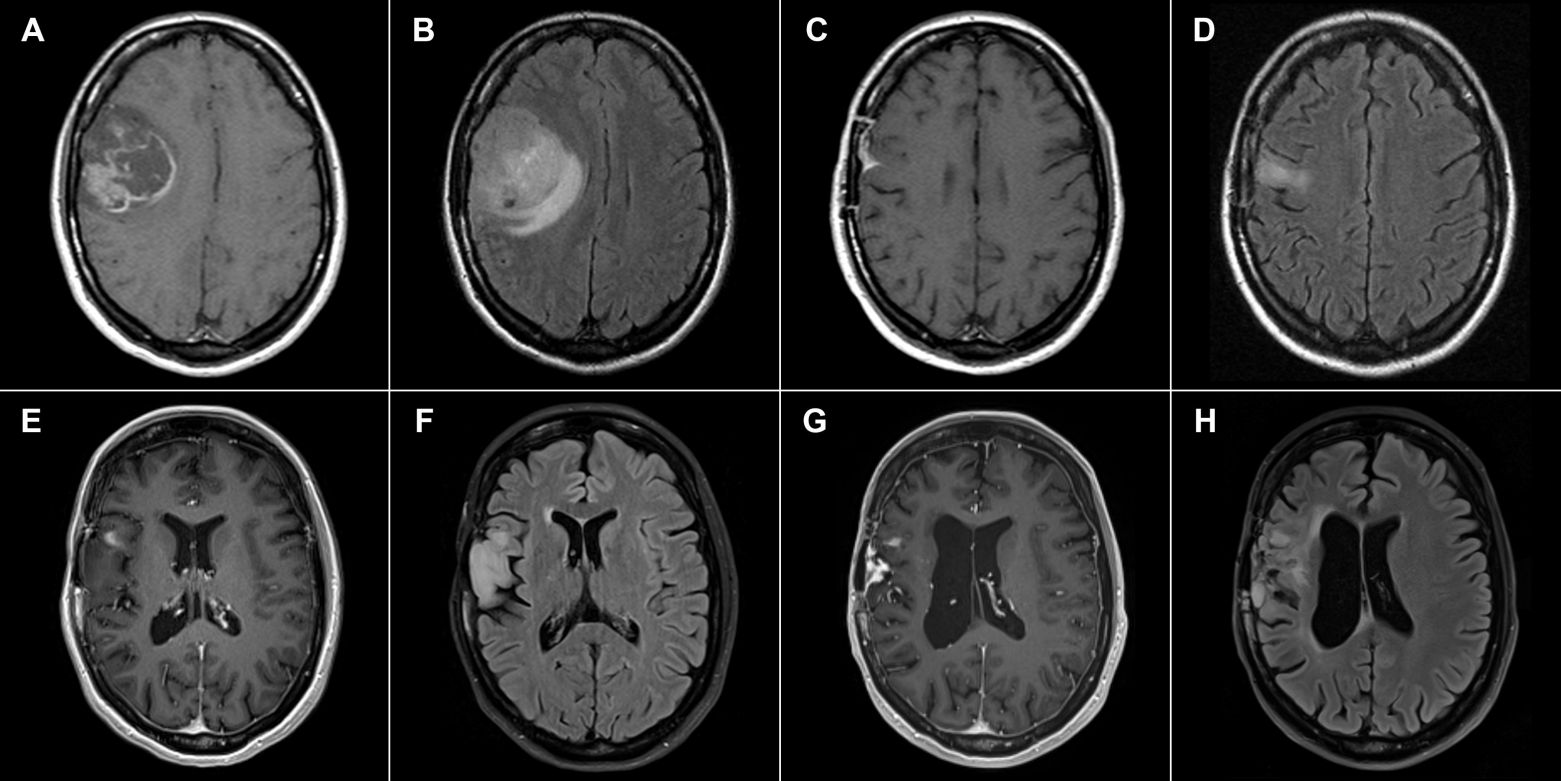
33-year-old female. (A) November 2005, 1 Tesla scanner: T1-weighted postcontrast spin echo preoperative image showing a GBM in the right central region. (B) Corresponding FLAIR image. (C) April 2006, 1 Tesla scanner, T1-weighted postcontrast spin echo postoperative image with a residual extra-axial enhancement. No enhancing residual tumor is present. (D) Corresponding FLAIR image. (E) 3 Tesla scanner, January 2021, T1-weighted postcontrast MP-RAGE sequence 181 months after surgery with signs of tumor recurrence in the right opercular region. (F) Corresponding FLAIR image. (G) 3 Tesla scanner, May 2022, T1-weighted postcontrast MP-RAGE sequence with a second recurrence in the same region. (H) Corresponding FLAIR image.
